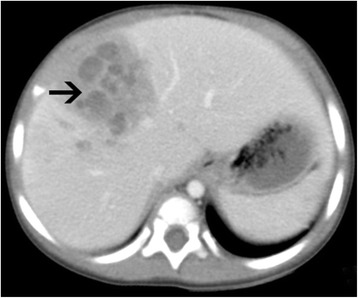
Computed tomography. Multiple low-density lesions in the right hepatic lobe (arrow),poorly circumscribed, with inhomogeneous enhancement, low-density necrosis, formation of visible partitions, and absent invasion of adjacent vessels
